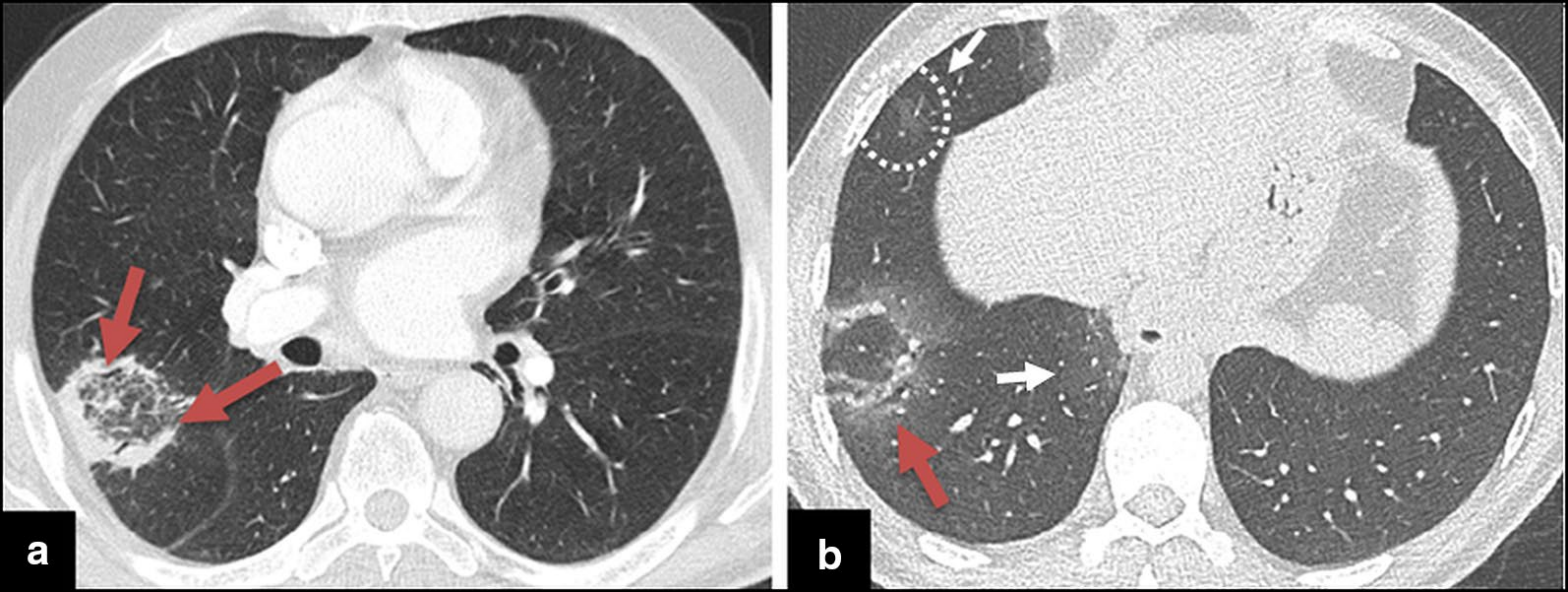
The reverse halo sign in cryptogenic organizing pneumonia and COVID-19 pneumonia. a Axial CT image showing organizing pneumonia presenting with reverse halo sign (arrows). b A rounded opacity with reverse halo sign and surrounding GGOs in the right lower lobe in COVID-19 pneumonia (arrow). GGOs are seen in the right middle lobe and medial right lower lobe (white arrows)
